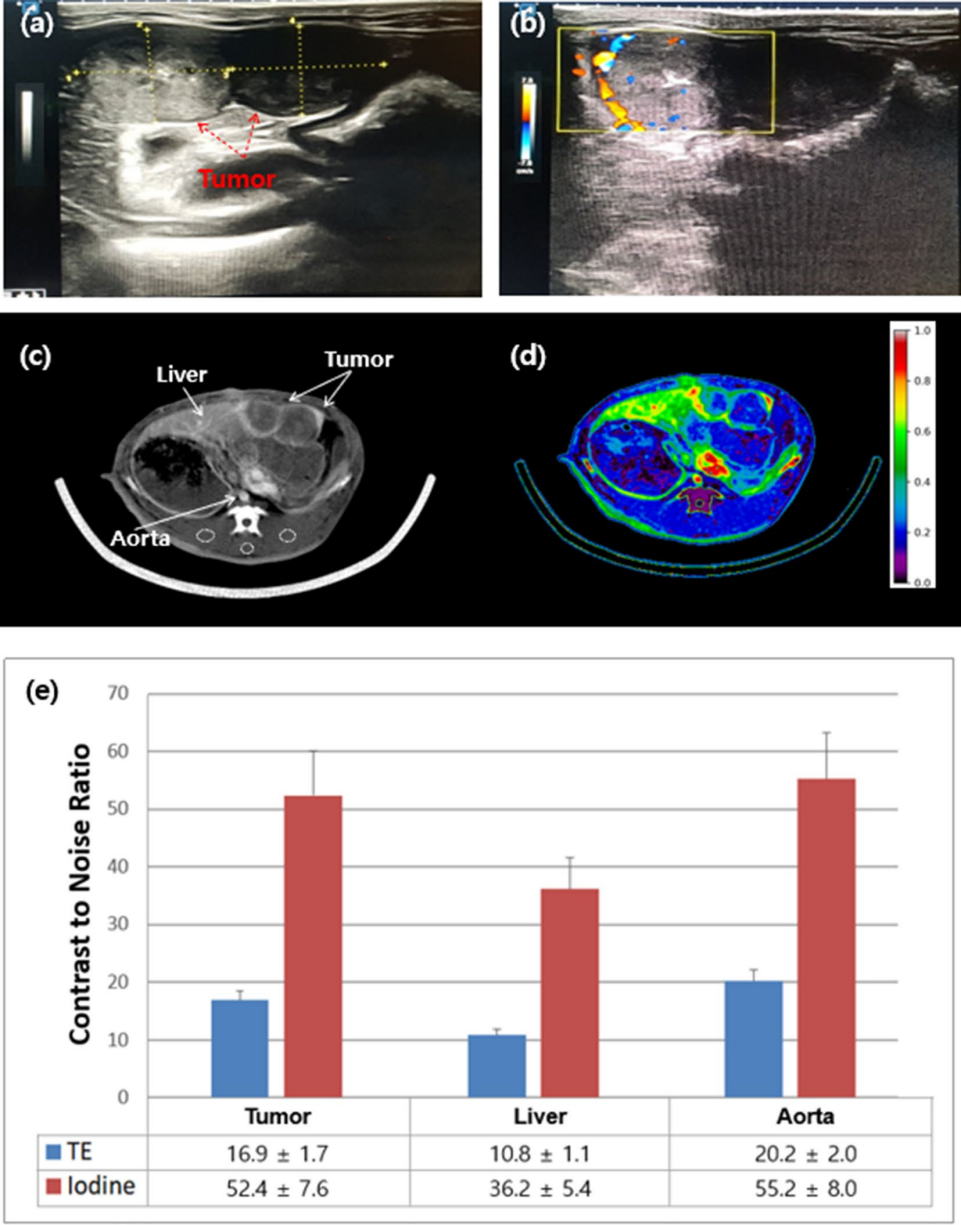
Rabbit with VX2 tumors located in the anterior part of the liver presented as (a) two-dimensional and (b) three-dimensional flow images produced via Doppler ultrasonography. (c) Contrast-enhanced axial image acquired at the total energy range and (d) iodine map image of the rabbit liver in the PCD CT system. The dotted circles are back muscles. 30–100 keV, 10 mAs, slice thickness = 2.5 mm, WW/WL [450/100]. (e) Contrast-to-noise ratio (mean ± SD) of the PCD CT system for the tumor, liver, and aorta in the total energy range (TE, 30–140 keV) of a rabbit acquired at an energy level in the range of 30–140 keV and the iodine map.
